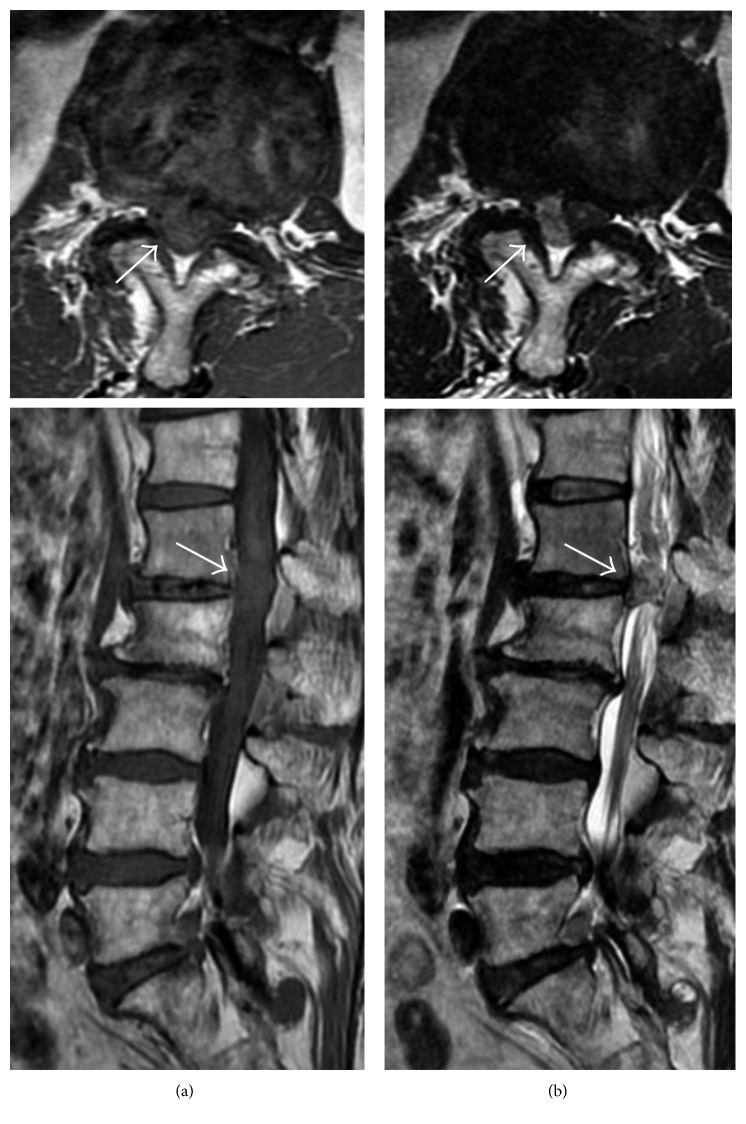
T1- and T2-weighted images from noncontrast magnetic resonance imaging: a mass is seen at the L1-2 level (arrow) with a low intensity on T1-weighted imaging (a) and a slightly high intensity on the T2-weighted image (b). The axial views demonstrate the mass occupying the right side of the spinal canal (arrow).
